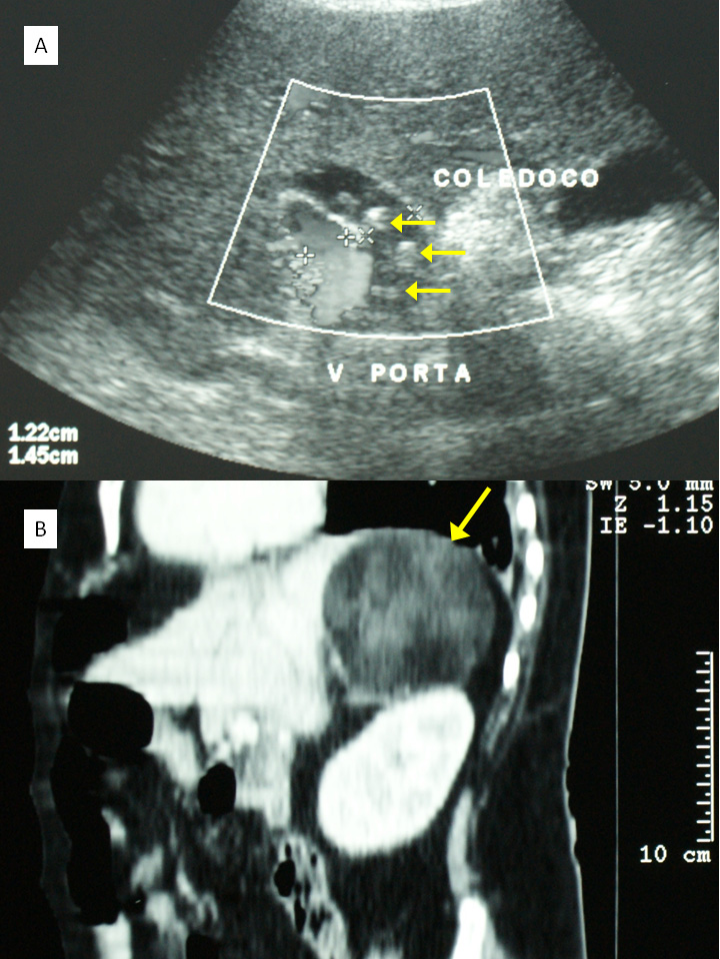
(A) Ultrasound aspect of dilated common bile duct with images suggestive of choledocholithiasis (arrows). (B) Computed tomography saggital view showing the mass in the right adrenal topography (arrow).
